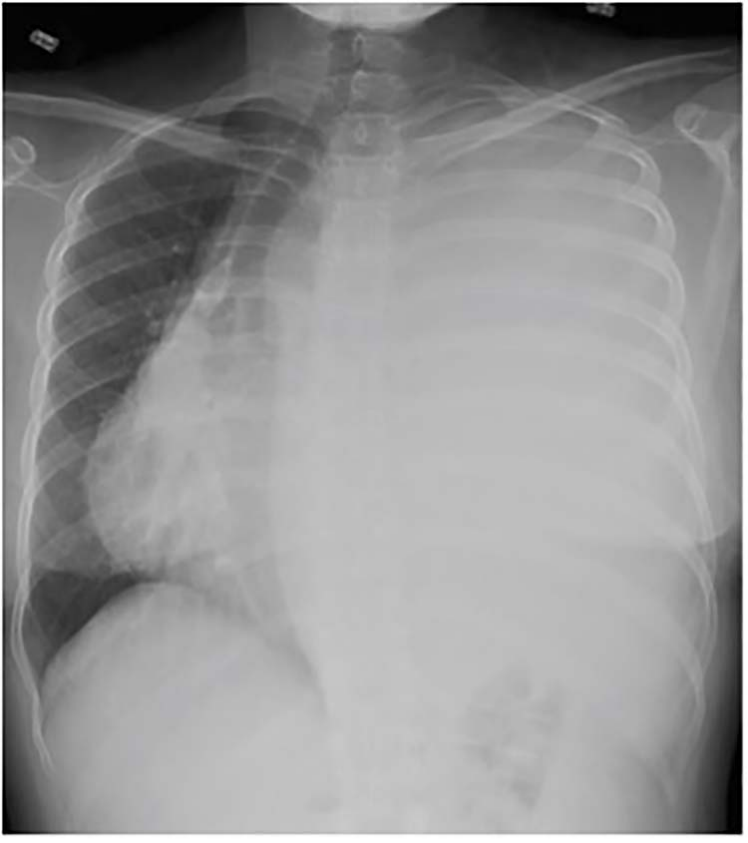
Frontal chest radiograph shows complete opacification of left hemithorax with significant mass effect.
